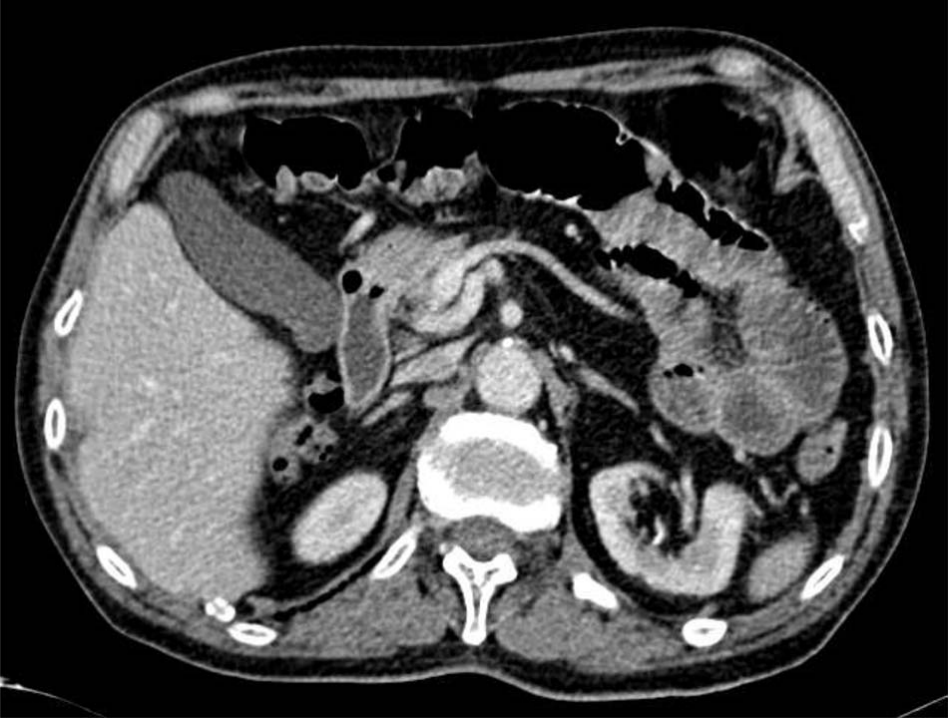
CT scan targetlike finding. Contrast enhanced CT scan of the abdomen demonstrates the typical multi-layered appearance of a small bowel intussusception. The intussusceptum is accompanied by a mesenteric fat and blood vessels and surrounded by the thick-walled intussuscipiens, the target-like finding, pathognomonic for intussusception.
